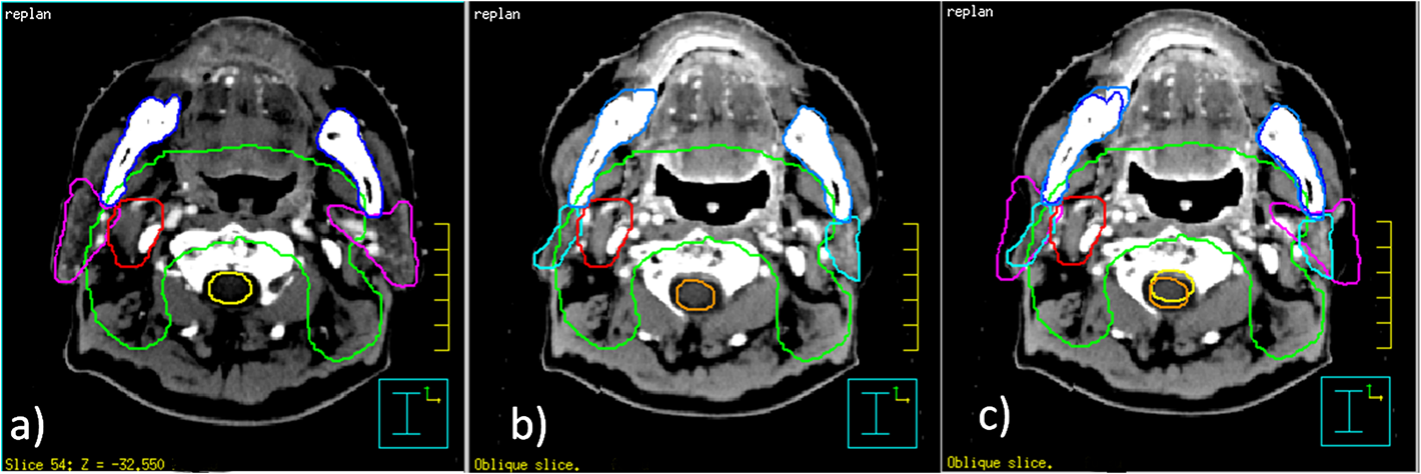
Volumetric changes between iCT and rCT. Volume changes between the initial planning CT and the rCT at the 23rd fraction; a) contours on the initial planning CT; b) contours on the rCT; c) contours on the initial planning CT projected on the rCT after image fusion.
